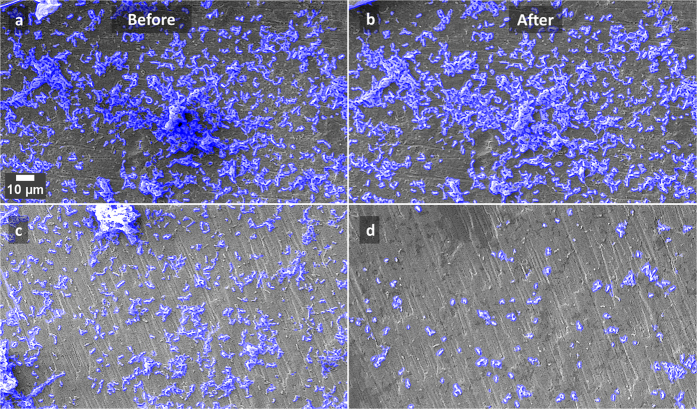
Examples of SEM images of the polished discs, before and after treatment with the ultrasonic scaler held 1 mm away for 30 s, at low power (a,b) and medium power (c,d). The blue overlay shows the automatic detection of bacteria.
